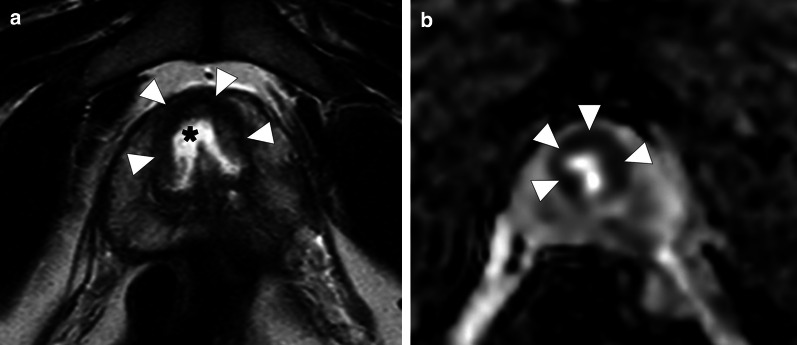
Prostatic involvement by granulomatosis with polyangiitis. A 31-year-old man with no known systemic disease presented with severe dysuria and hematuria. Serum PSA levels were also increased. Axial T2-weighted MR image a of the prostate demonstrates heterogeneous ill-defined, mostly hypointense lesion (arrowheads) in the prostate apex. A small cystic cavity appears in the central part of the lesion (asterisk). Axial ADC map (b = 800 s/mm^2^; 1.5 T) b shows diffusion restriction in the peripheral part of the lesion, suggestive of increased cellularity (arrowheads). Fusion guided transrectal biopsy revealed granulomatous prostatitis consistent with granulomatosis with polyangiitis
